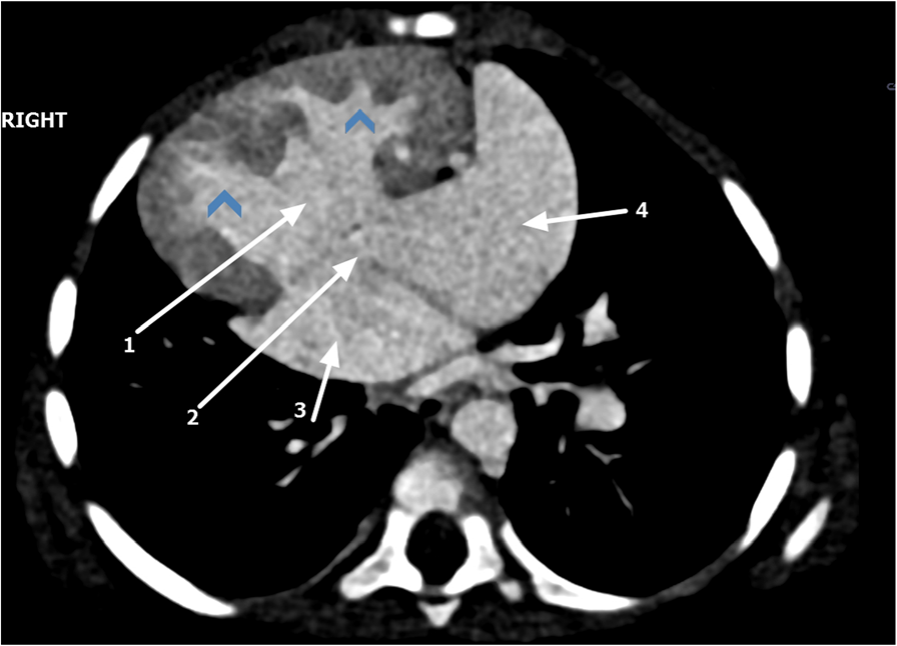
Cardiac computed tomography axial view showing dextroposition of the heart, ventricular septal defect (1), atrial septal defect (2), double right atrium (3, 4), and transposed ventricles (*arrowheads*)
